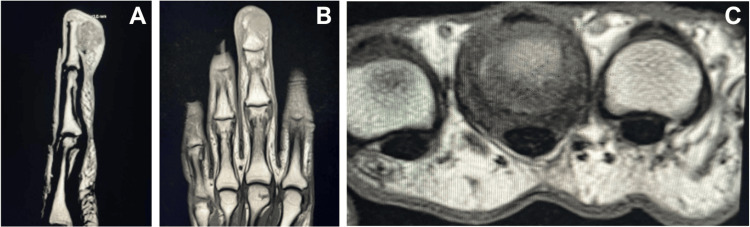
Preoperative MRI Preoperative MRI of the left long finger showing the enhancing subcutaneous mass along the volar aspect with erosive changes, displayed in the (A) sagittal, (B) coronal, and (C) axial planes.
